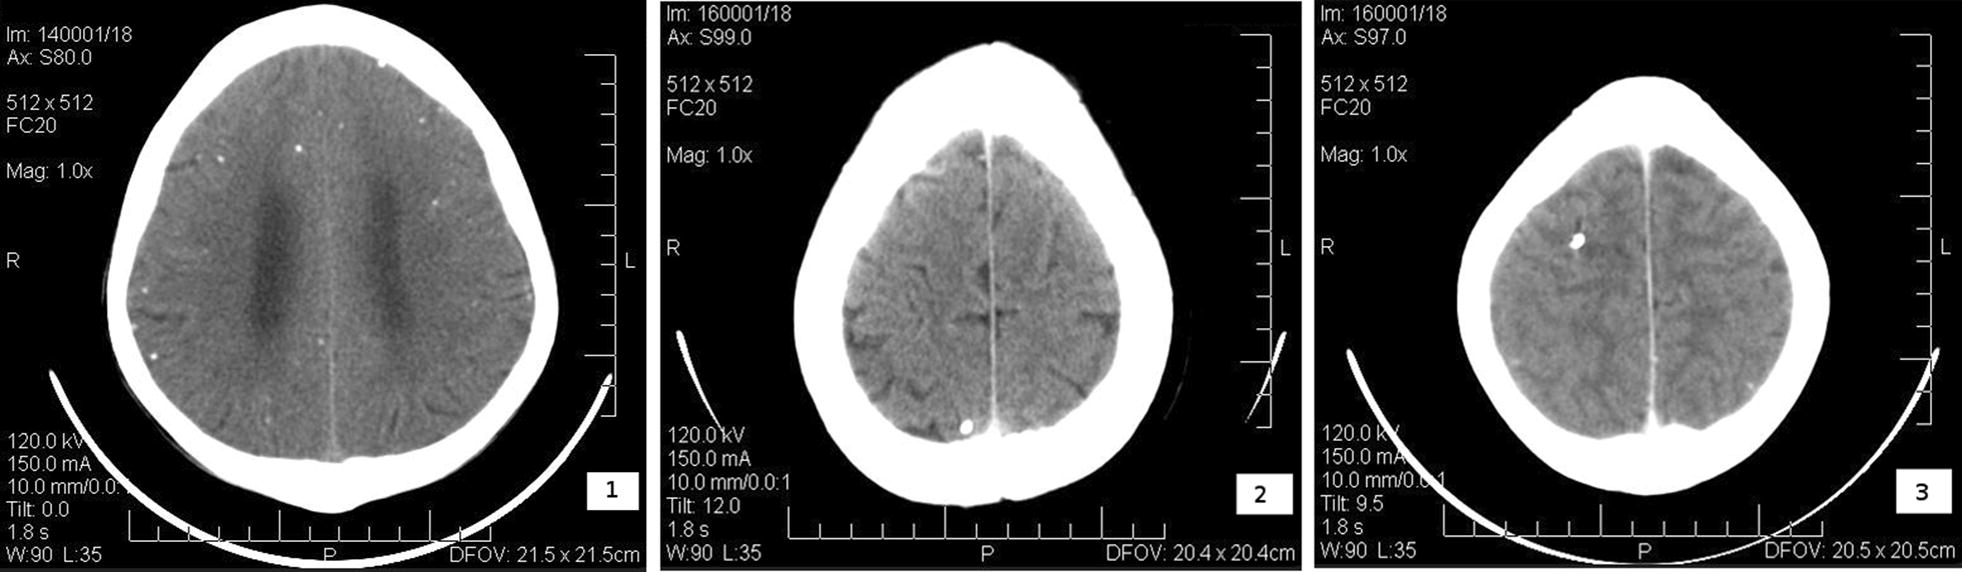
CT scans of three of the HCC sero-positive cases showing widely and diffusely spread calcifications in the brain^1^; calcification in the right occipital lobe^2^ and calcification in the left temporal-frontal lobe^3^
